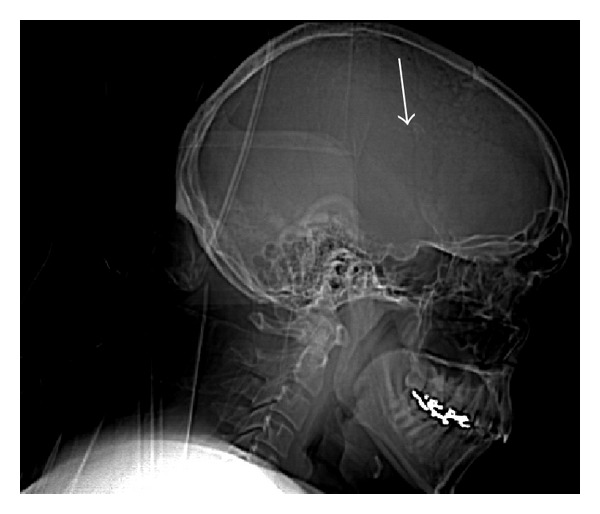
Lateral radiograph of the skull demonstrating a focus of calcification in the frontoparietal region (arrow).
